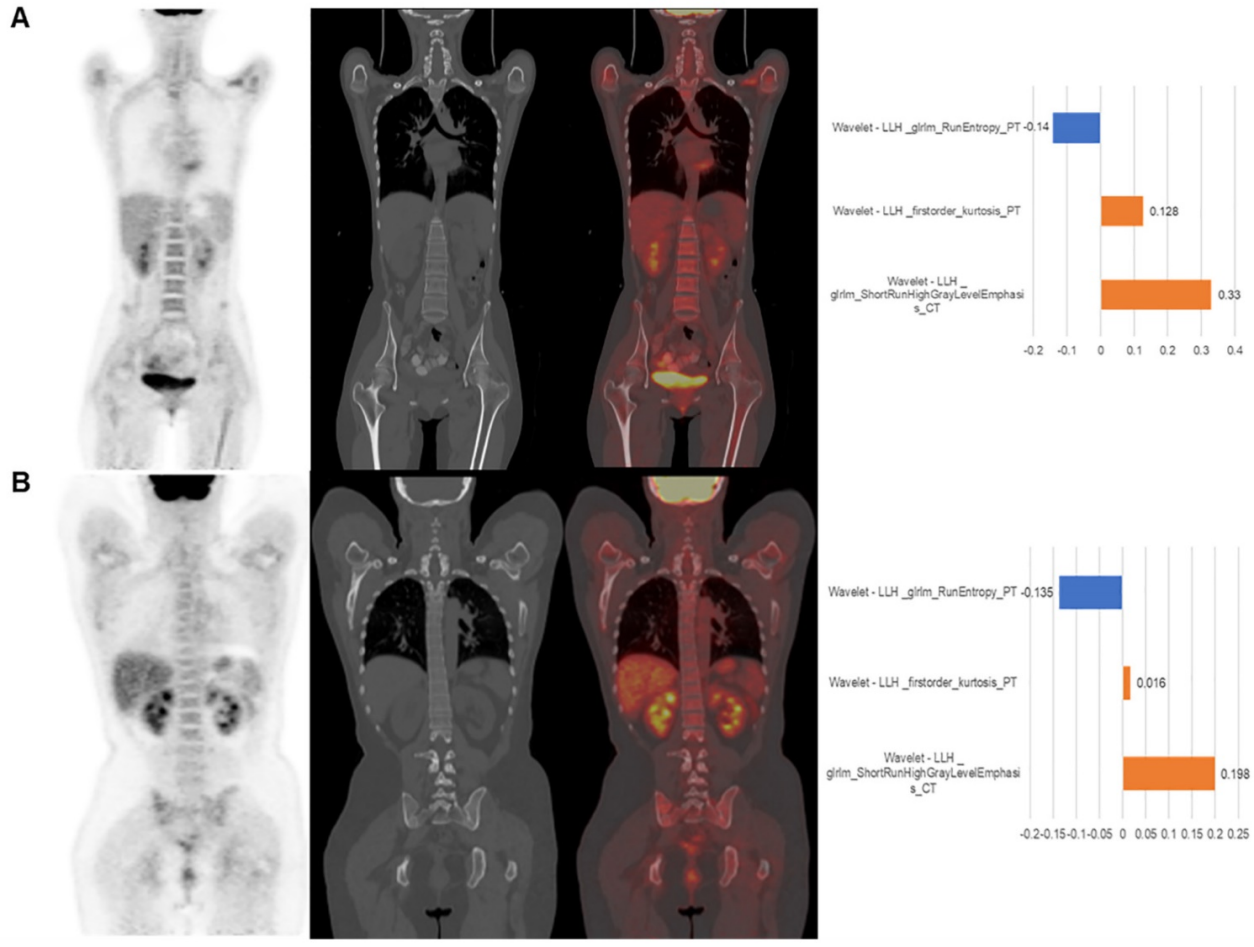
The patient displayed on panel (A) was BMB negative. The machine learning model correctly predicted it. The value of three features were -0.03, -0.57 and -0.23, respectively. The visual diagnosis was false positive. The patient displayed on panel (B) was BMB positive. The machine learning model correctly predicted it. The value of the three features were -0.48, -0.34 and -0.28, respectively. The visual diagnosis was false negative. From left to right, coronal PET, CT, fusion image and the approximated features weights from LIME interpretation.
